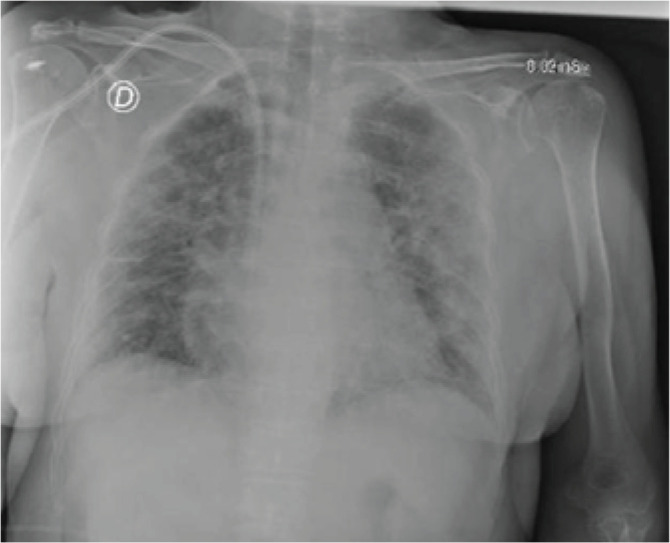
Anteroposterior chest X-ray (AP), showing mild cardiomegaly, symmetric pulmonary vasculature, and basal subpleural reticulation, with mixed opacities in both pulmonary fields in a ground glass pattern, most of them, occupying the alveolar spaces, probably as result of alveolar haemorrhage.
